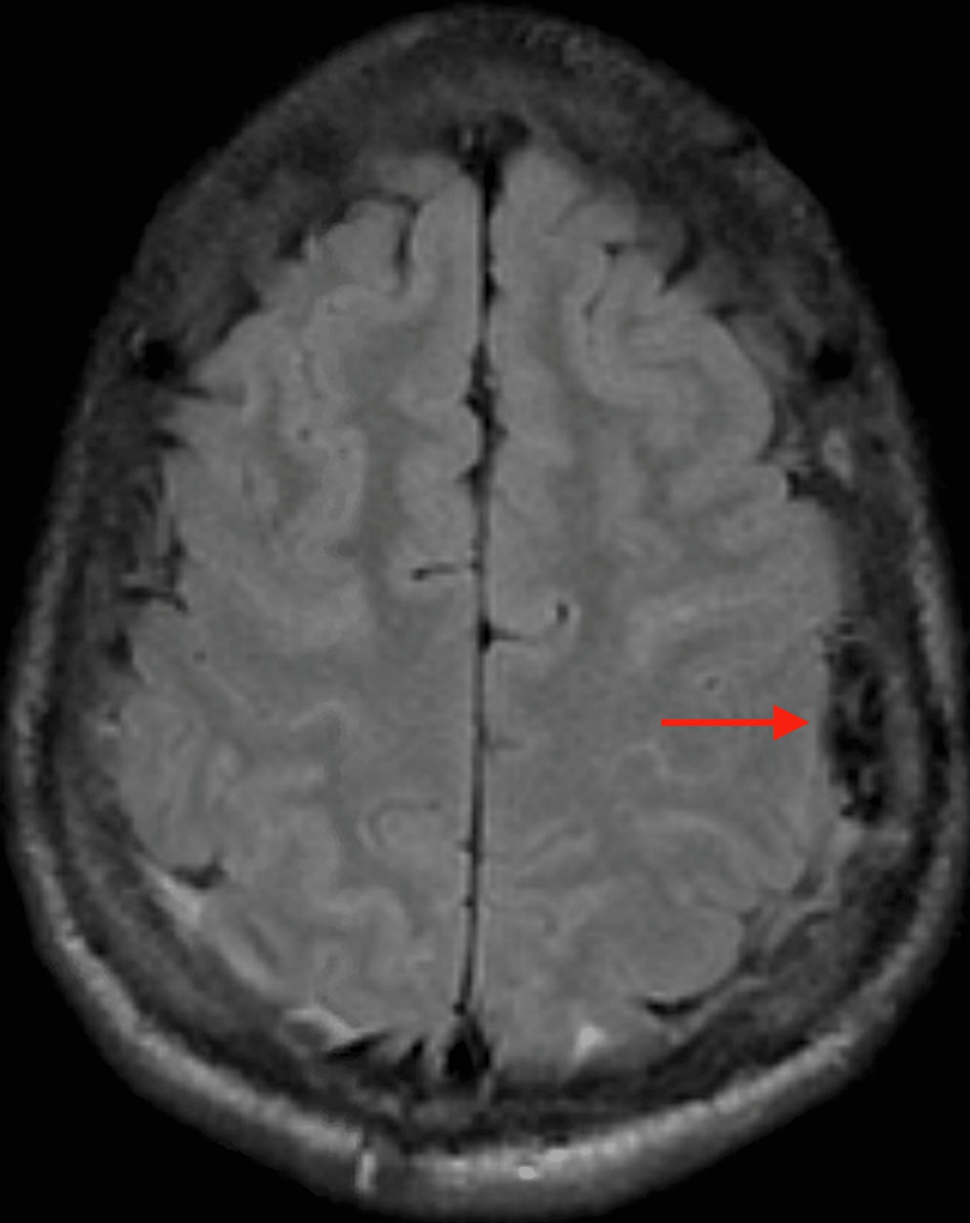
Axial T2 flair MRI showing left parietal subdural hematoma (arrow)
